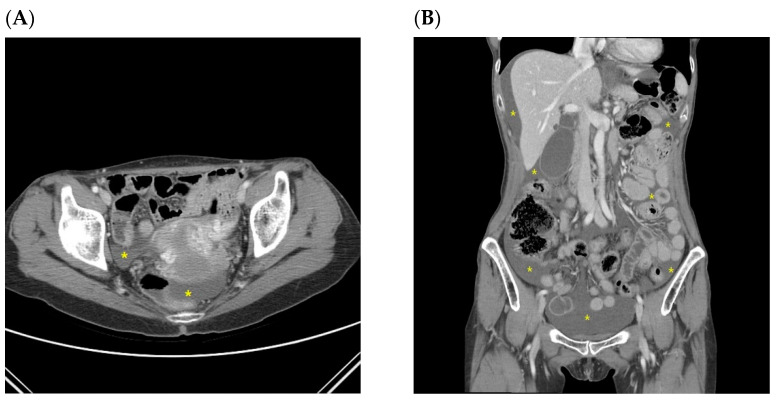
CT images of a patient on initial recurrence of malignant ascites without other CT findings related to peritoneal seeding, later confirmed by cytology. A small volume of pelvic ascites fluid was observed at first appearance (A). Follow-up CT (B) showed an increased volume of ascites fluid. Yellow stars indicate ascites.
